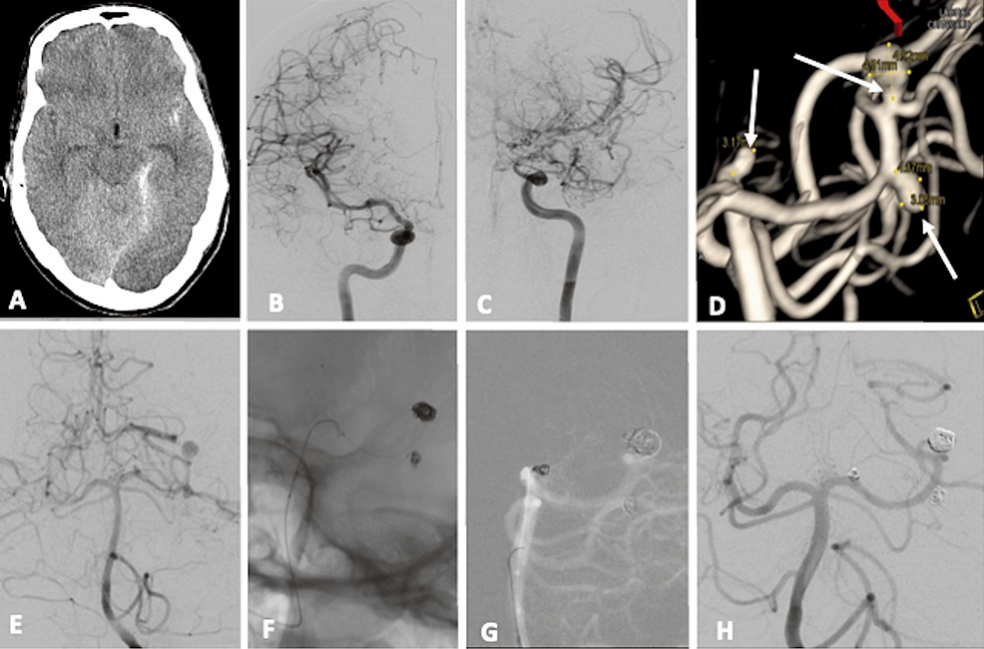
Noncontrast CT and DSA images pre- and post-coiling of a patient with multiple aneurysms with MMD (Case 5). Axial CT image (A) showed a subarachnoid bleed in the left Sylvian fissure and left tentorium cerebelli. B&C revealed a narrowing of bilateral supraclinoid ICA, A1 anterior cerebral artery (ACA), and left M1 middle cerebral artery (MCA). A 3D angiogram (D) and left vertebral artery (VA) angiogram (E) showed three flow-related aneurysms, one each in P1, distal P2, and P3 posterior cerebral arteries (PCA) (white arrows). All three aneurysms were coiled under balloon protection (F-H), with the exclusion of an aneurysm and good filling of left PCA on the control angiogram.
